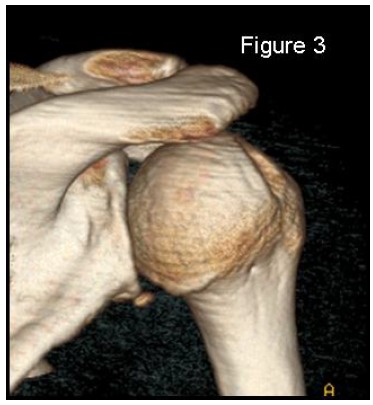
3D-CT is the gold standard to evaluate and quantify the humeral head bone defect. 54x59mm (150 x 150 DPI).
